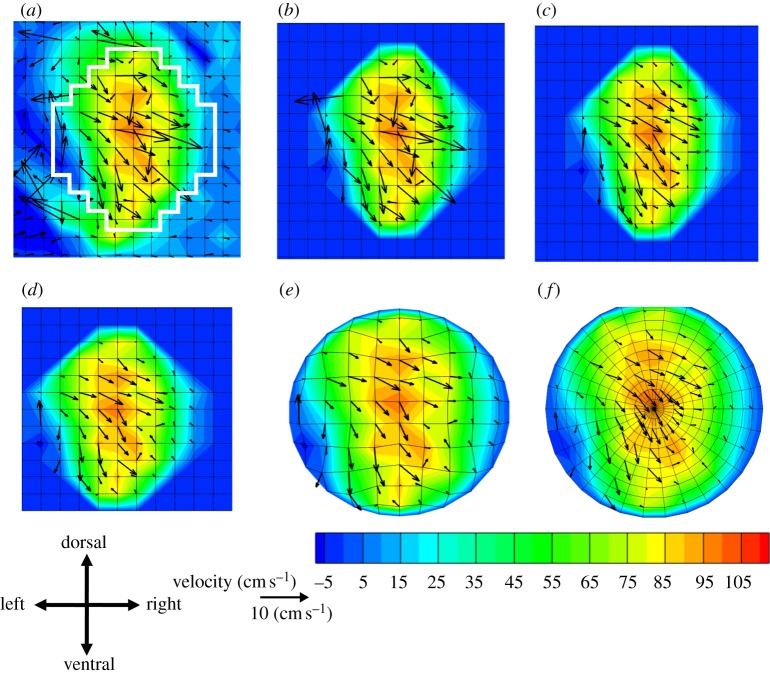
MRI data at different stages of processing. (*a*) Unwrapped, offset data with vessel mask shown in white, (*b*) masked data, (*c*) corrected for spurious vectors, (*d*) corrected for vessel movement, (*e*) warped to circle and (*f*) interpolated to mesh with constant radial and azimuthal spacing. Colour contours of the through-plane velocity and in-plane velocity vectors are shown.
